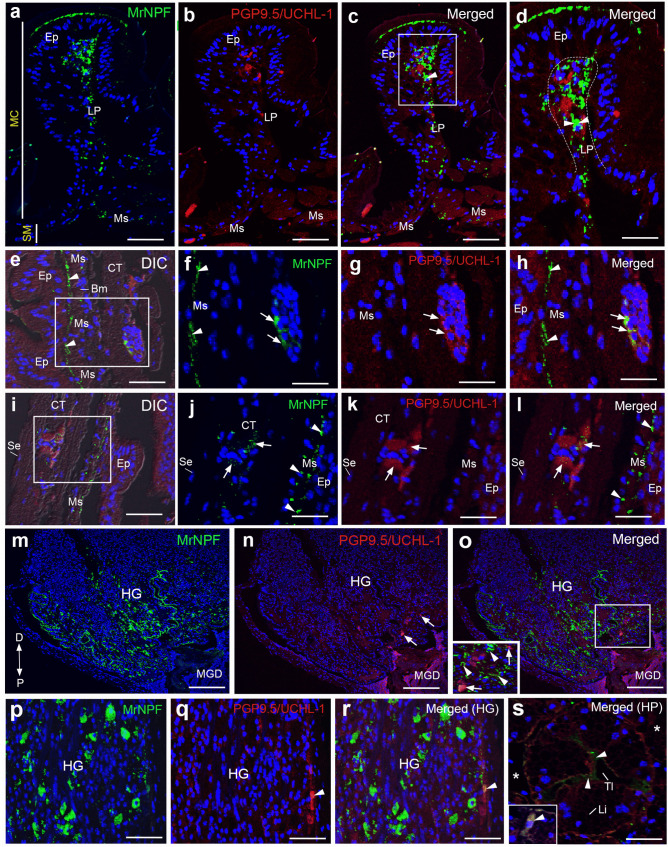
Representative images showing the presence and colocalization of MrNPF (green) and PGP9.5/UCHL-1-like-ir (red) immunolabelling in the FG, MG, HG, and HP of female *M. rosenbergii* (at least *n* = 15 female prawns), while nuclei were counterstained with DAPI (blue). a–d Representative images demonstrating the expression of MrNPF-ir and PGP9.5/UCHL-1-like-ir, respectively (a and b). Strong PGP9.5/UCHL-1-like-ir is observed in the Lp (white arrowheads) in the PY. Colocalization of intense MrNPF-ir and PGP9.5/UCHL-1-like-ir in the Lp layer of the PY region is seen (c and d). e, i Intense MrNPF-ir and PGP9.5/UCHL-1-like-ir in MG. The figures e and i are merged with DIC images. f, j MrNPF-ir is observed in some cells in the Ms (white arrowheads) and the CT layer (white arrows), while strong PGP9.5/UCHL-1-like-ir is expressed in a few cells of the CT layer (white arrows) of MG (g, k). h, l Colocalization of a few MrNPF-ir and PGP9.5/UCHL-1-like-ir cells is demonstrated in the CT layer of MG (white arrows). m, n Images showing MrNPF-ir in Vi and G of HG, whereas intense PGP9.5/UCHL-1-like-ir was detected in the Vi parts of HG (white arrows). o Colocalization of a few MrNPF-ir and PGP9.5/UCHL-1-like-ir cells in the Vi is observed (inset in o, white arrows shows colocalization, while white arrowheads only exhibit Mr-NPF-ir). p, q The prevalence of MrNPF-ir in the G part of HG (white arrowhead), while little colocalization of a few MrNPF-ir and PGP9.5/UCHL-1-like-ir cells in the HGG region (white arrowhead) (r). s Little colocalization of MrNPF-ir and PGP9.5/UCHL-1-like-ir in the HP (white arrowheads), and a few colocalized cells are observed (inset). Bm, basement membrane; CT, connective tissue; Ep, epithelial cell; HG, hindgut; Li, lipid droplets; Lp, lamina propria; MC, mucosa layer; MGD, midgut distal part; Ms, muscle; Se, serosa; SM, submucosa layer; Tl, tubular lumen; *, interlobular area. Scale bars 100 µm (a, b, c, e, i, m, n, and o), 50 µm (d, f, g, h, j, k, l, p, q, and r), and 25 µm (inset of o)
